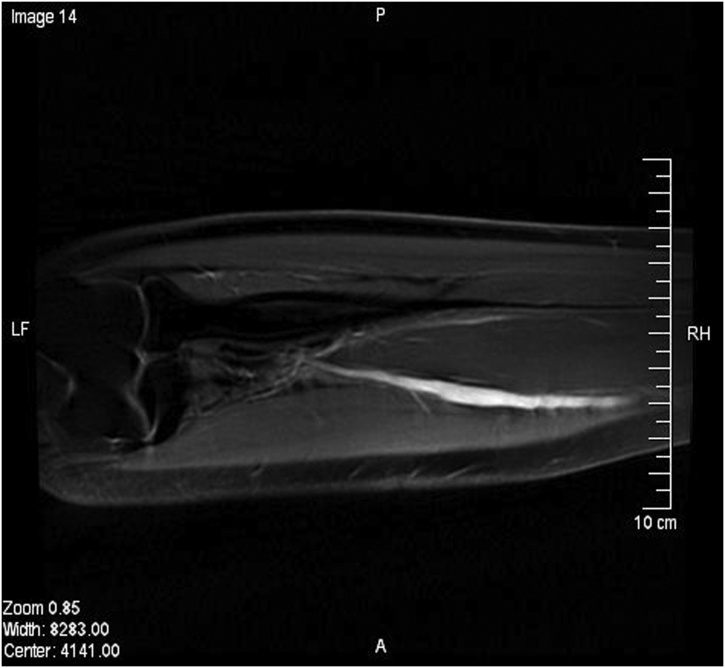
Longitudinal MRI of the forearm showing ulnar artery aneurysm.
